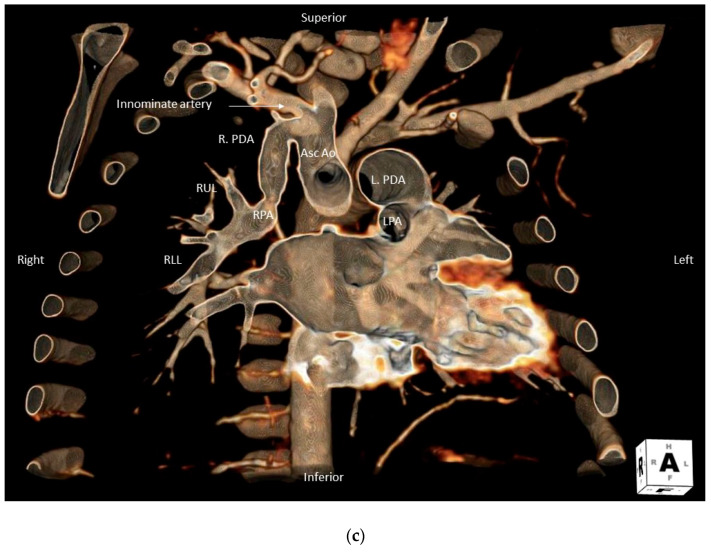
(a) Abnormal “5-vessels” noted in a standard 3VT fetal echocardiography view. Viewed from right to left, these vessels include right pulmonary artery (1), right superior vena cava (2), aorta (3), main pulmonary artery (4), and left superior vena cava (5). (b) Fetal echocardiogram sagittal plane color Doppler image showing the anomalous origin of the right pulmonary artery (RPA) from an aortic arch branch. Asc Ao, Ascending aorta; Right pulm vein, right pulmonary vein. (c) Computed tomography angiography (CTA) coronal view showing the right pulmonary artery origin from a right ductus arteriosus from the base of the innominate artery. R. PDA, right-sided patent ductus arteriosus; RPA, right pulmonary artery; RUL, right upper lobe branch; RLL, right lower lobe branch; Asc Ao, ascending aorta; L. PDA, left-sided patent ductus arteriosus; LPA, left pulmonary artery.
